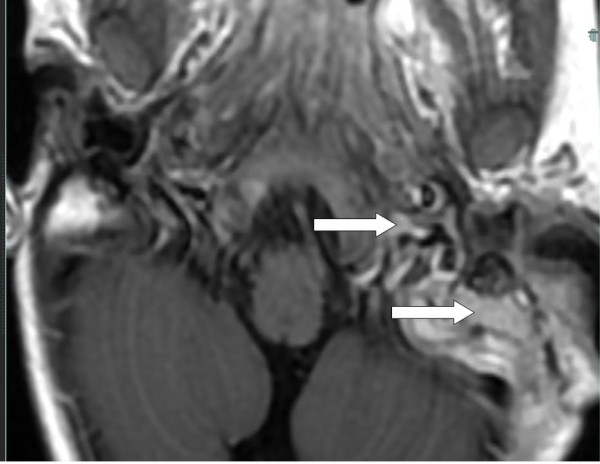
MRI horizontal section T1 + Gadolinium. Arrows show areas with enhancement in left pars petrosa.
